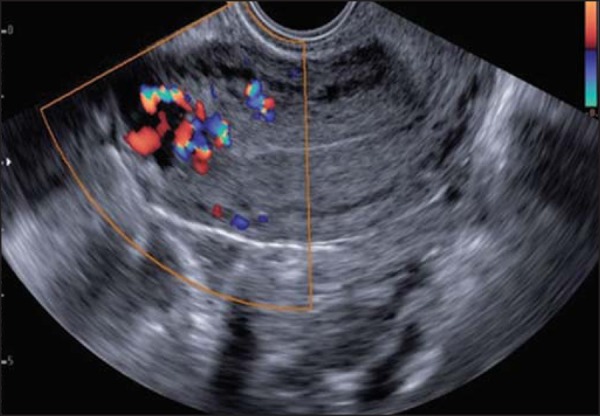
Transvaginal ultrasound, acquired during post-molar pregnancy
